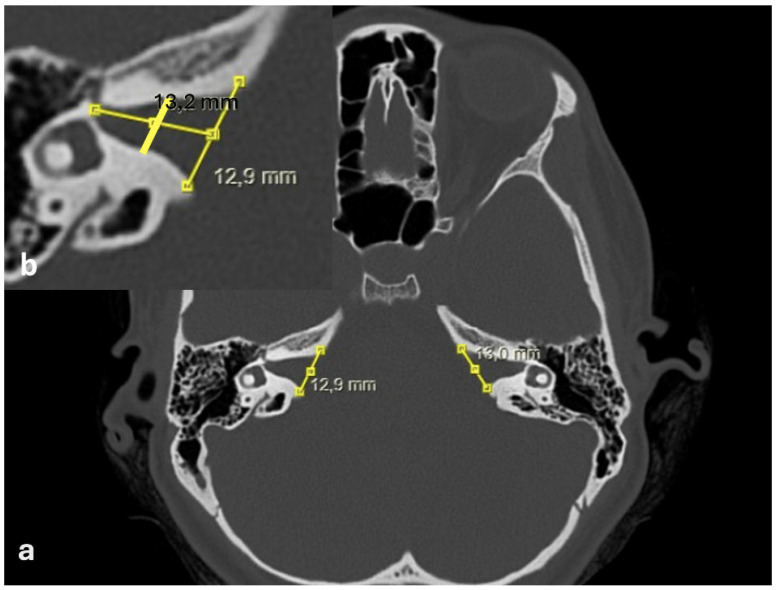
(a) Axial temporal bone CT image showing bilateral IAM inlet diameters (mm). (b) Measurement of right IAM length and mid-diameter (mm), with the line connecting the inlet midpoint to the most lateral point of the IAM.
